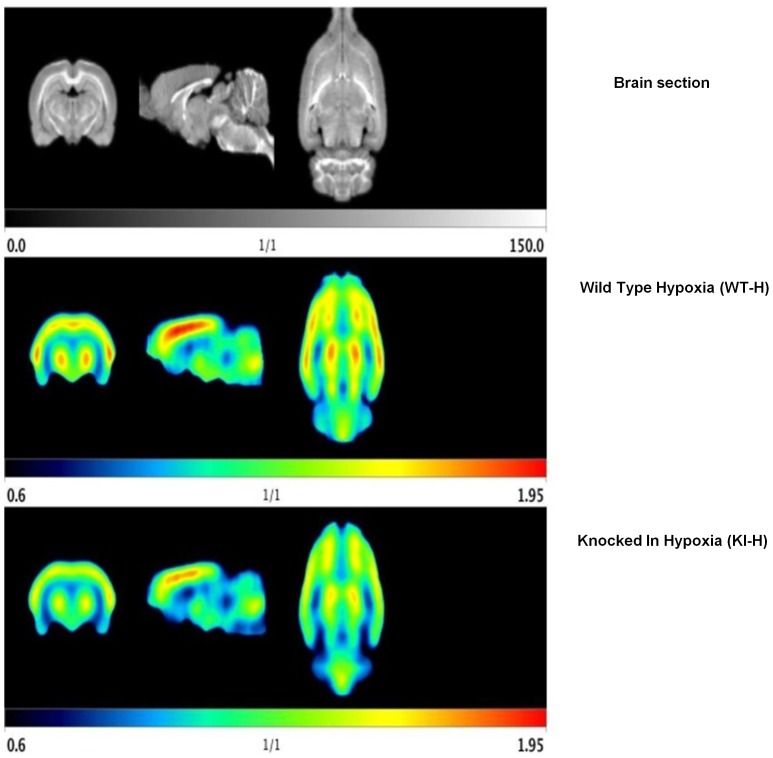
Brain PET scan with an FDG standardized uptake value, showing a cross and longitudinal sections of brain uptake.
